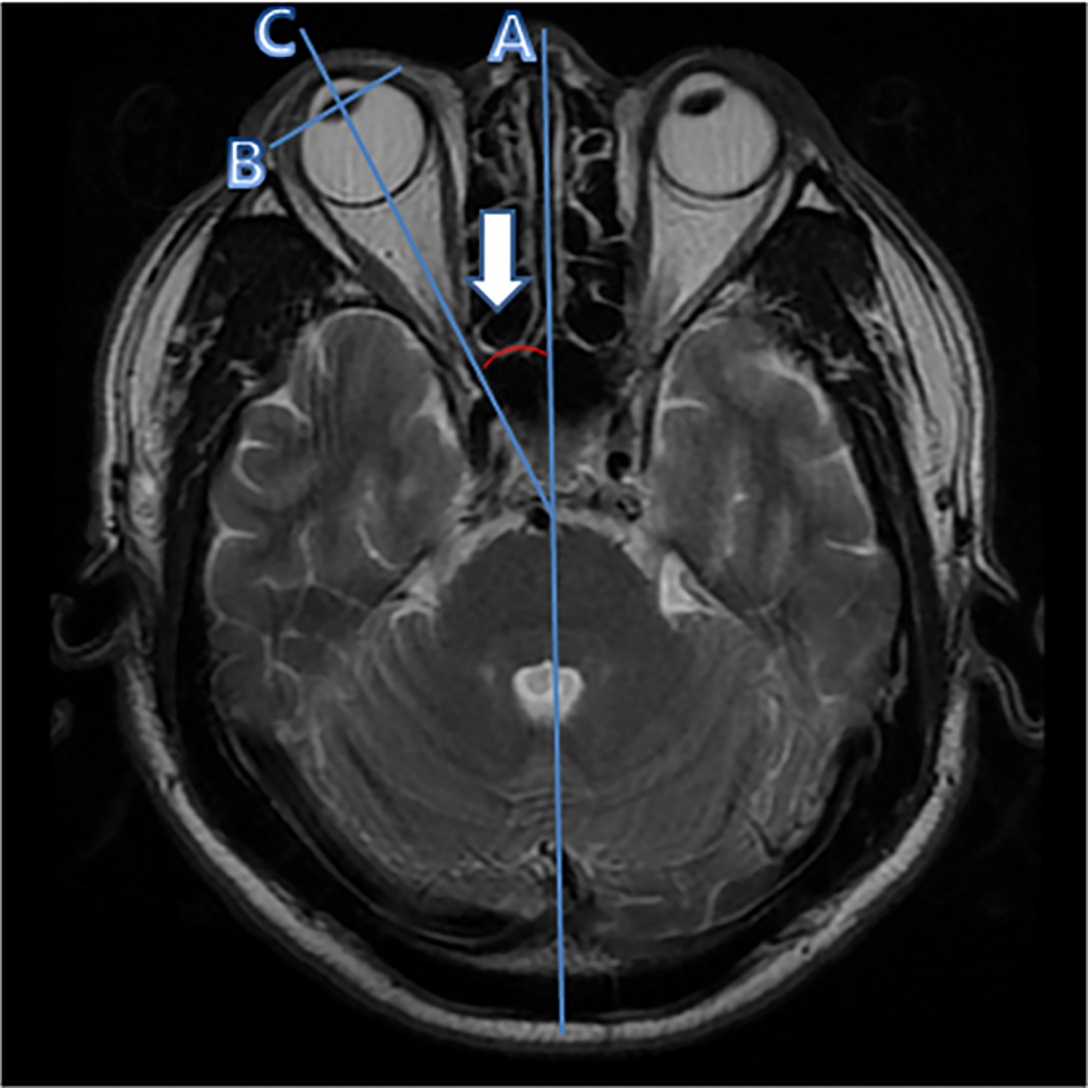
Ocular deviation (OD) measurements in T2-weighted axial images of brain MRIs. Three lines were drawn to measure OD values in T2-weighted axial images. The first line (A) was drawn on the midline of the nasal structures. The second line (B) connected both ends of the lens. The third line (C) was drawn perpendicular to line B. We measured the angle formed by the intersection of lines A and C (arrow).
